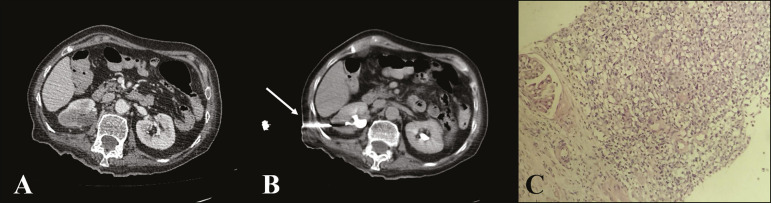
A: CT scan of the abdomen, axial section, showing an ill-defined urothelial lesion in the middle third of the right kidney. B: Performance of the CT-guided biopsy (arrow) using the coaxial technique after injection of the contrast agent in the excretory phase. C: Anatomopathological examination revealed invasive urothelial carcinoma.
